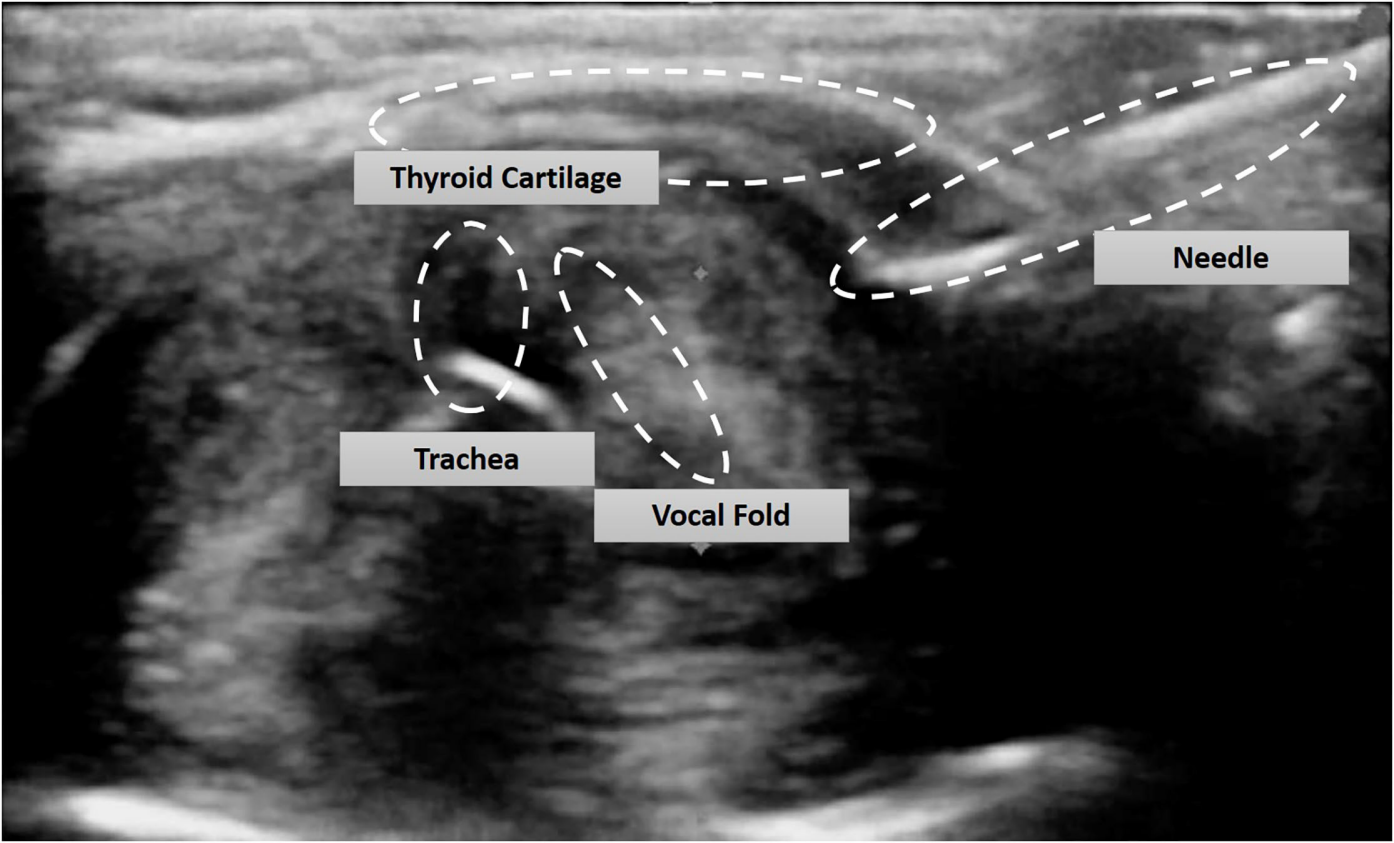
Axial ultrasound of the larynx with in-axis placement of the 18 G needle.
